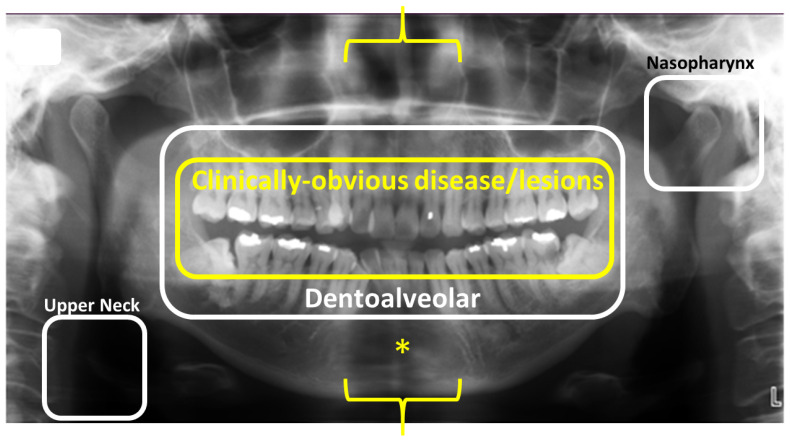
The focus of most dentists when using the dental panoramic radiograph is the dentoalveolar apparatus, the contents of the white box, whereas the yellow box denotes only those structures available to direct dental clinical examination. The areas on the DPR where one would look for nasopharyngeal lesions or calcified carotid artery calcification in the upper neck are indicated by white squares. The yellow brackets denote the anterior sextant, the display of which on the DPR is compromised by both the superimposition of the vertebral column and the narrow focal trough anteriorly. The yellow asterisk is in the center of a ‘radiolucency’, appearing like a cyst or neoplasm, which is an artifact derived from a combination of the vertebral superimposition and narrow focal trough adapted from Figure 9.1 in Reference [12]; MacDonald, Wiley-Blackwell 2020.
